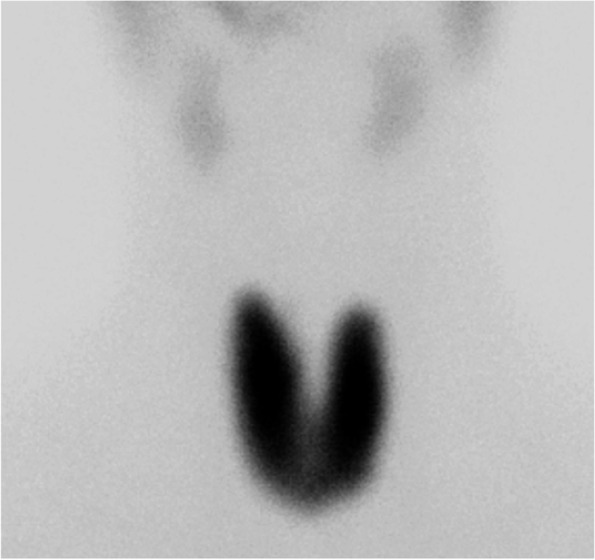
^99m^Tc scintigraphy. Diffuse uptake in the thyroid gland was revealed
